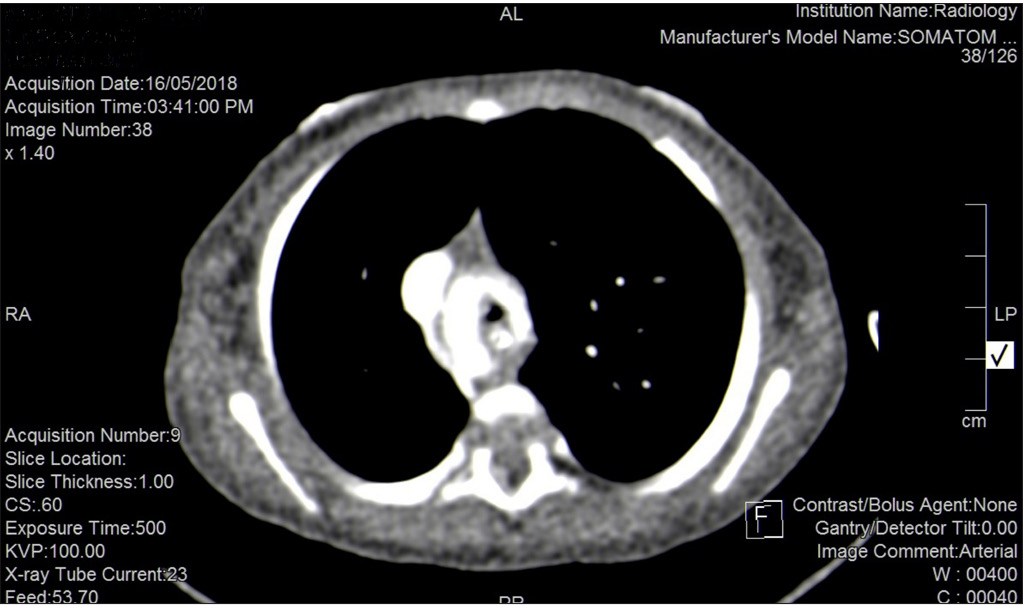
Axial computed tomography angiogram demonstrating an
